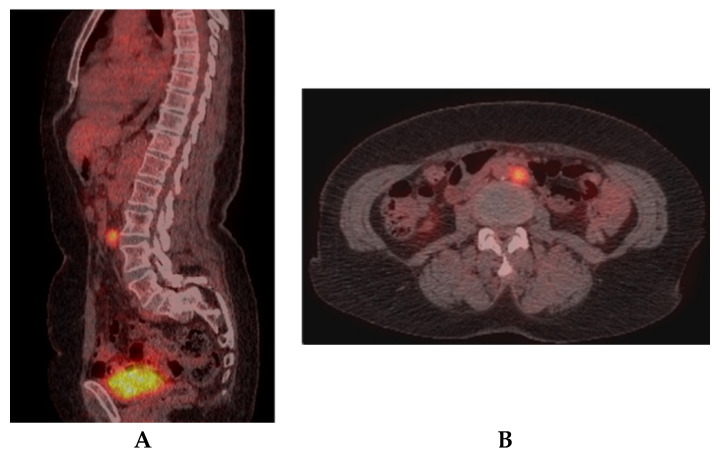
F18-FDG PET/CT scans in 2017 after treatment, evaluating the CA 125 increase during the follow-up: sagittal section abdominal level (A), pelvic level (B) showing tracer high uptake in left abdominal latero-aortic lymph node (SUVlbm Max—4.43), with recurrence after complete remission in 2016.
